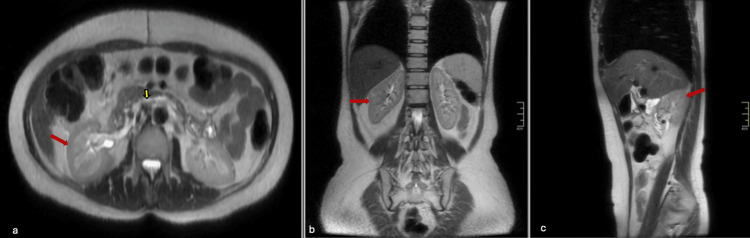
Follow-up non-contrast abdominal MRI Complete resection of the lesion. The right kidney (red arrow in (a-c)) has returned to its normal position within the renal fossa, showing normal size and enhancement pattern. The inferior vena cava (yellow arrow) is located in its normal retroperitoneal space adjacent to the aorta. No residual mass or abnormal soft tissue density is observed. MRI: magnetic resonance imaging
